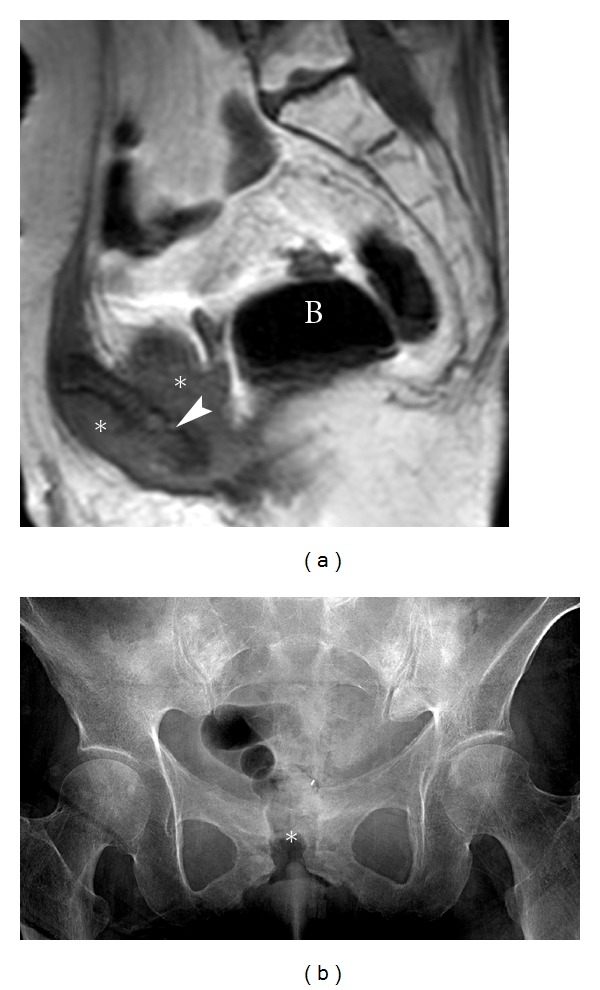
Pubic osteomyelitis. A 70-year-old female, with a history of previous surgery and radiotherapy for carcinoma of the cervix, presented with an open wound over the symphysis pubis. The clinical concern was the possibility of local tumor recurrence in the pubic symphysis region. The patient was subsequently diagnosed as having osteomyelitis. Sagittal T1 MRI (a) shows a soft tissue mass (asterisk) with underlying destruction of the symphysis pubis (arrowhead). Note the presence of air in the bladder (B) from a vesicovaginal fistula. Biopsy and culture proved the changes were due to osteomyelitis, rather than tumor recurrence. AP Radiograph (b) showing cortical irregularity and destruction in the region of pubic symphysis with widening of the joint (asterisk). Gas is projected within the symphysis pubis and adjacent soft tissues in keeping with the known vesicovaginal fistula.
